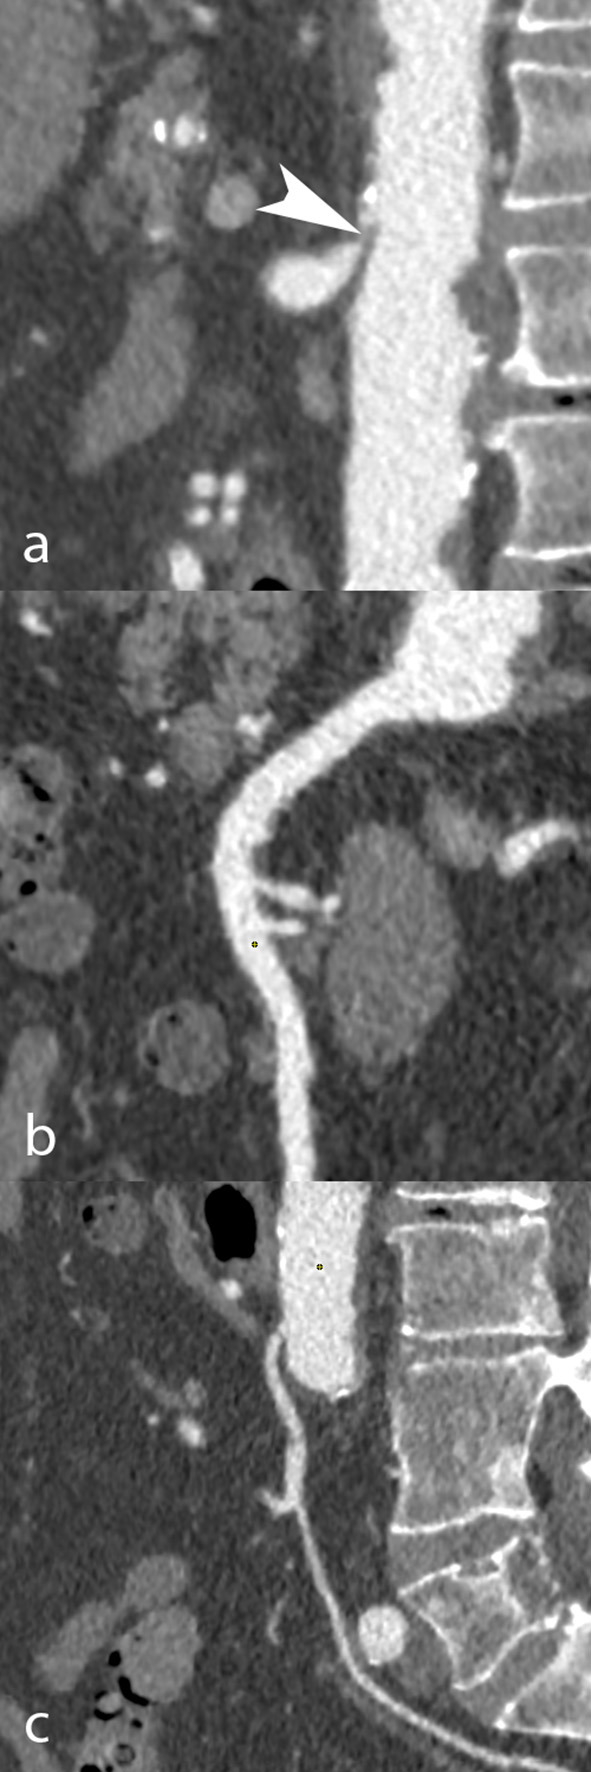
CT angiography in an 85-year-old man scheduled to undergo TAVI. Curvilinear reconstructions show the coeliac trunk (a), superior (b), and inferior mesenteric artery (c). While the coeliac trunk was occluded due to the combination of an arcuate ligament and a mixed plaque (white arrowhead), the mesenteric arteries had no occlusive disease.
